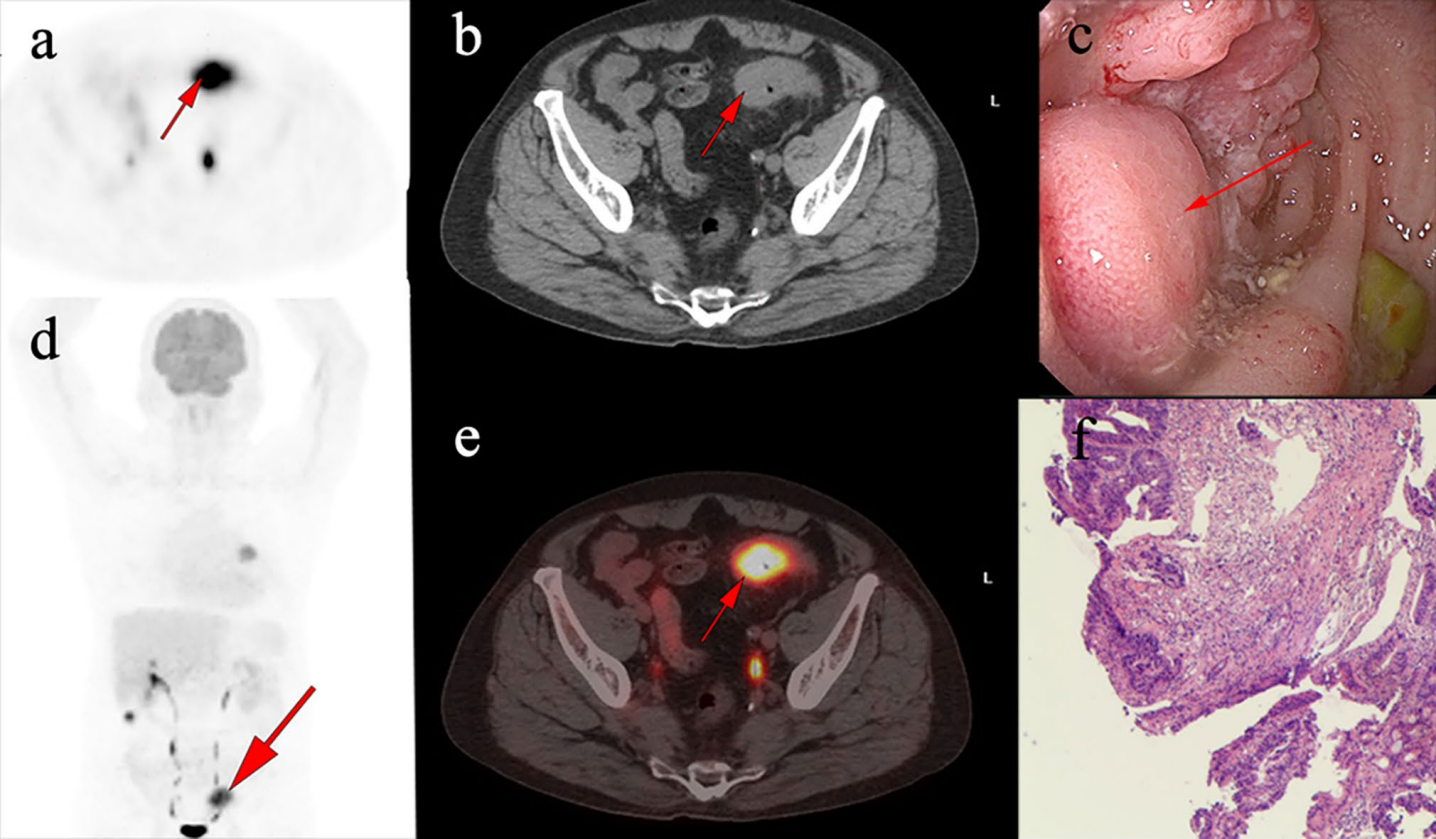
A 69-year-old man underwent ^18^F-FDG PET/CT for left lung carcinoma. Incidental focal ^18^F-FDG uptake was found in the sigmoid colon. The patient underwent colonoscopy and pathology examination. a-b, d-e Abnormal ^18^F-FDG PET/CT uptake in the sigmoid colon. c shows a sigmoid colon polyp with a diameter of 3.4 cm. f shows the pathological pattern of high-grade intraepithelial neoplasia
